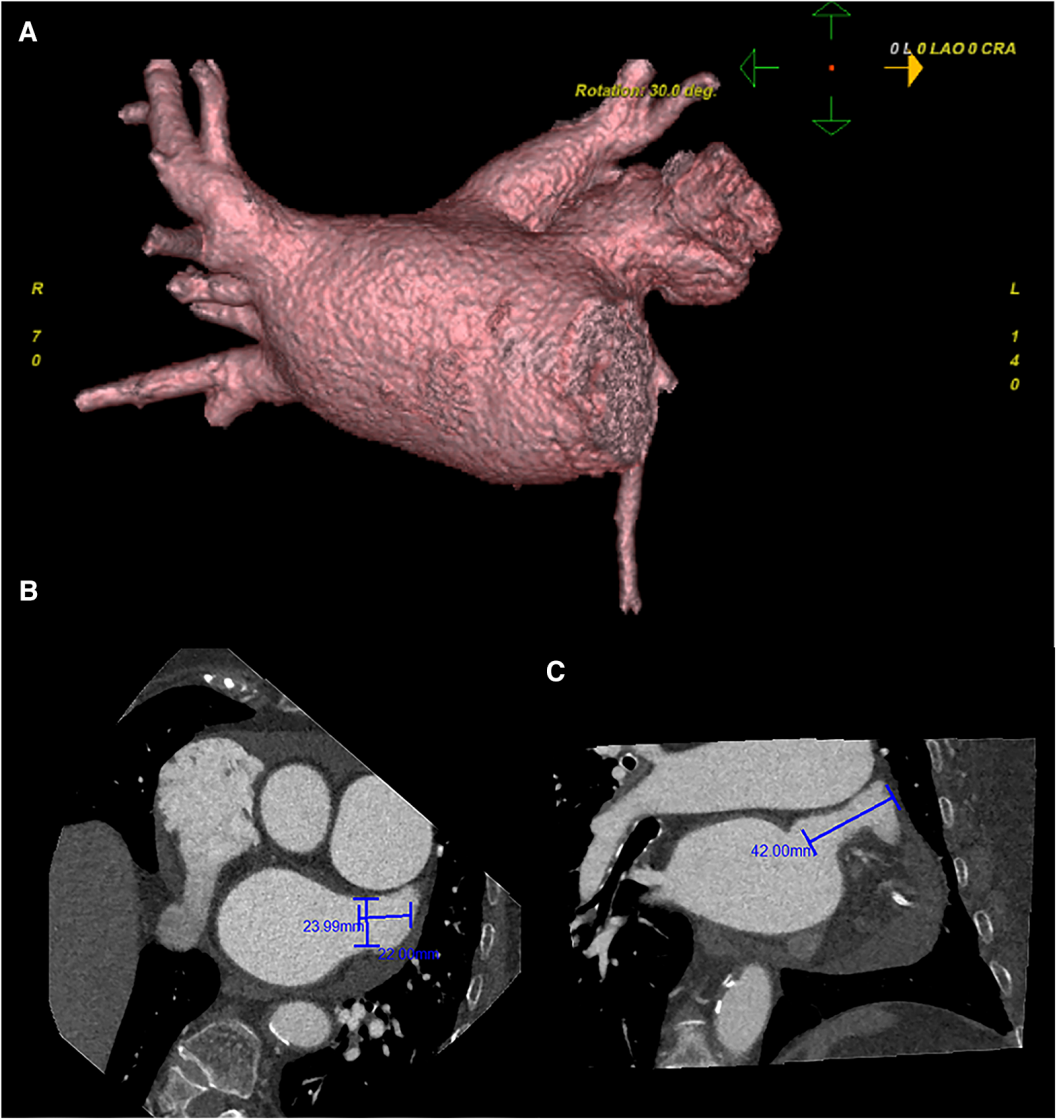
Preoperative cardiac CT angiography reveals a complex left atrial appendage with a large branch, no clear filling defect in the left atrial appendage and left atrium, and no diverticulum and accessory atrial appendage was identified in the left atrium (A) the cross-sectional image of the LAA orifice (B) the long diameter was about 2.4 cm, the short was about 2.2 cm. Oblique view (C) the maximum depth of the left atrial appendage branch was about 4.2 cm. Pericardial effusion.
